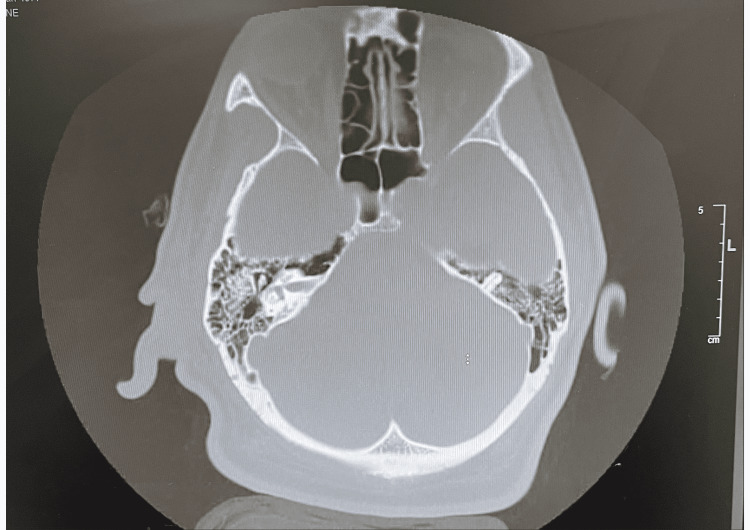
CT ears without contrast showed gross soft tissue edema about the pinna of the right ear and within subcutaneous fat at the base.
